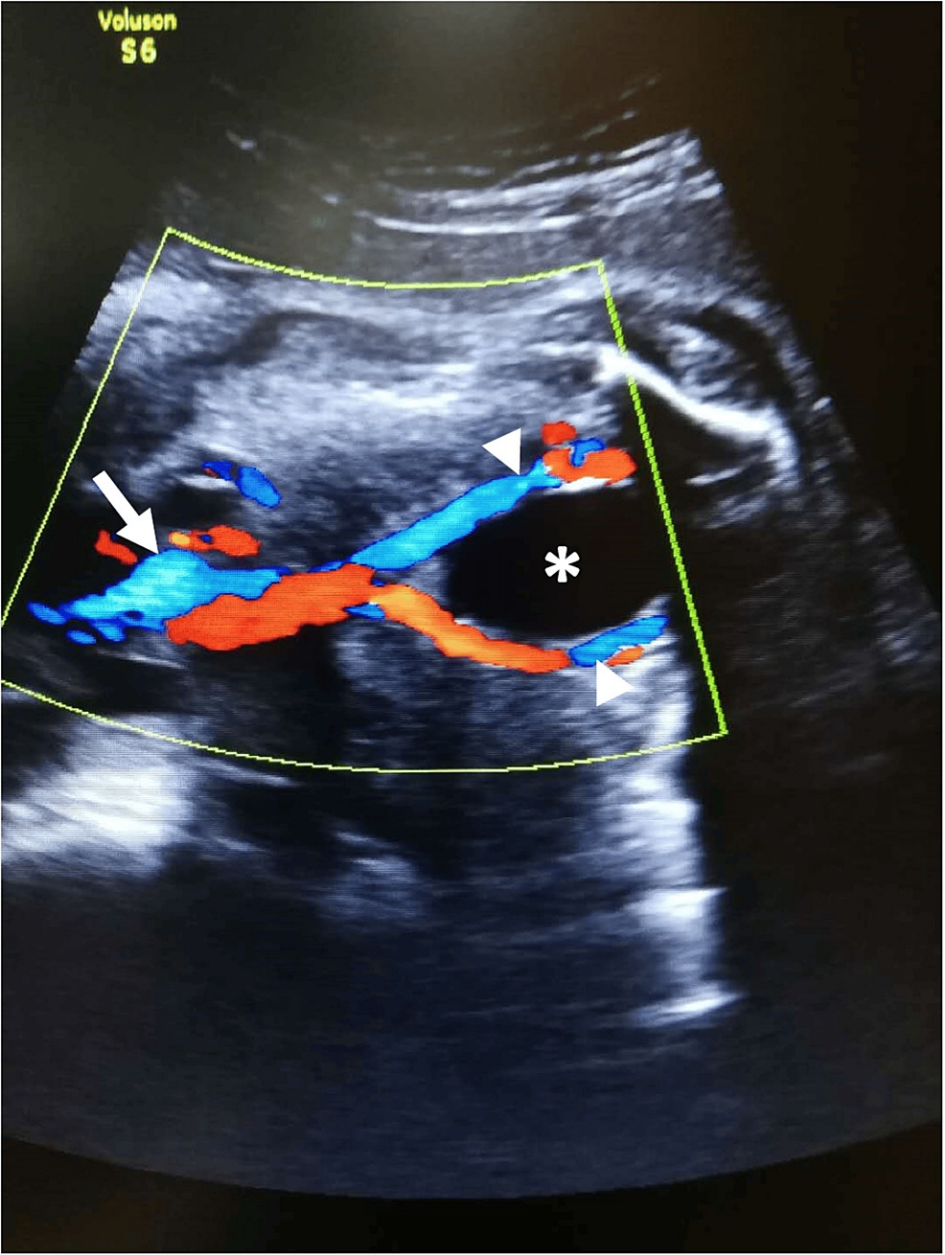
Ultrasound scan with Doppler study The routine ultrasound scan cross-sectional views of ultrasound images at the bladder level (asterisk) showed one umbilical vein (arrow) and two umbilical arteries (arrowhead).
